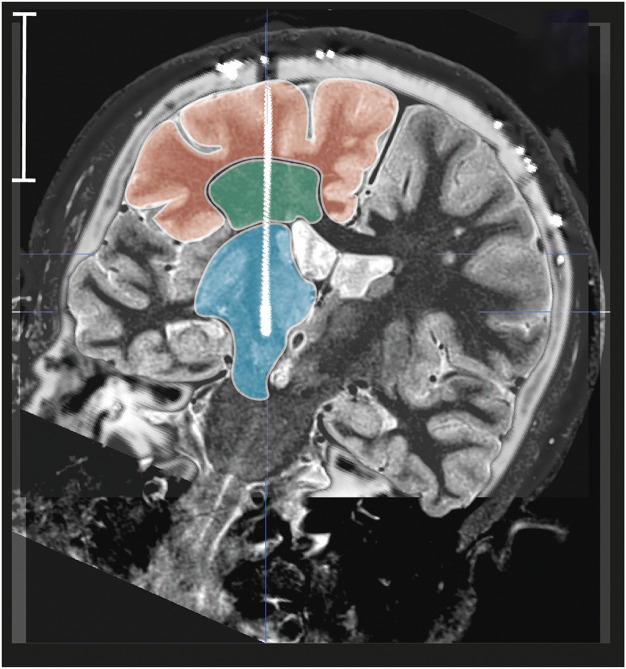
Tissue layers. Example of overlay of postoperative CT scan and preoperative MRI (reconstructed pseudo coronal slice along the right electrode) showing the three tissue layers: the superficial gyration layer (red), the intermediate white-matter layer (green), and the deep brain layer (blue); white vertical bar = 50 mm.
